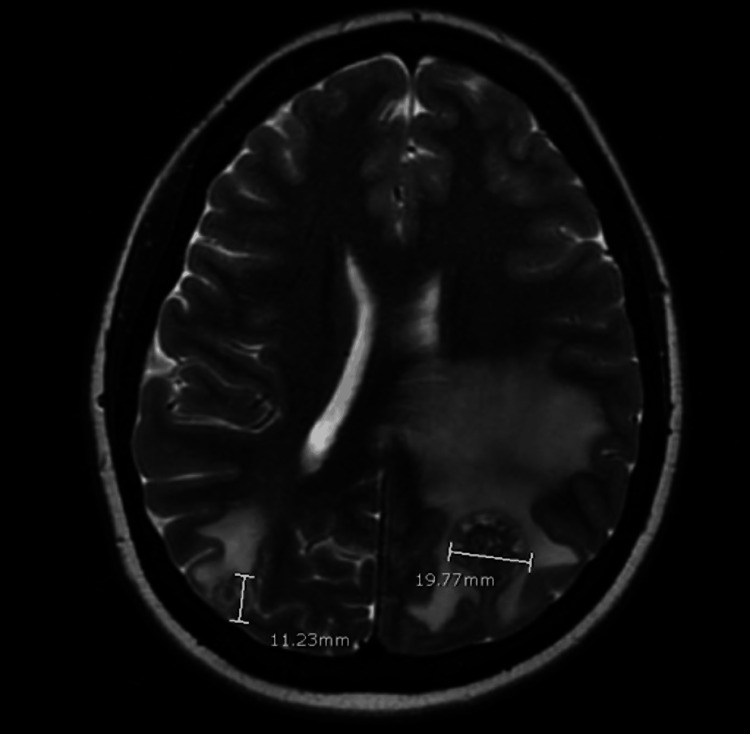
Axial T2-weighted MRI showing measurements of metastases: a right posterior parietal 1.1 x 1.2 x 1.0 cm lesion and a left parietal lobe lesion measuring 2.1 x 1.9 x 2.0 cm with left frontoparietal vasogenic edema surrounding the largest lesions, causing effacement of the left lateral ventricle and minimal focal rightward midline shift measuring 4.2 mm
